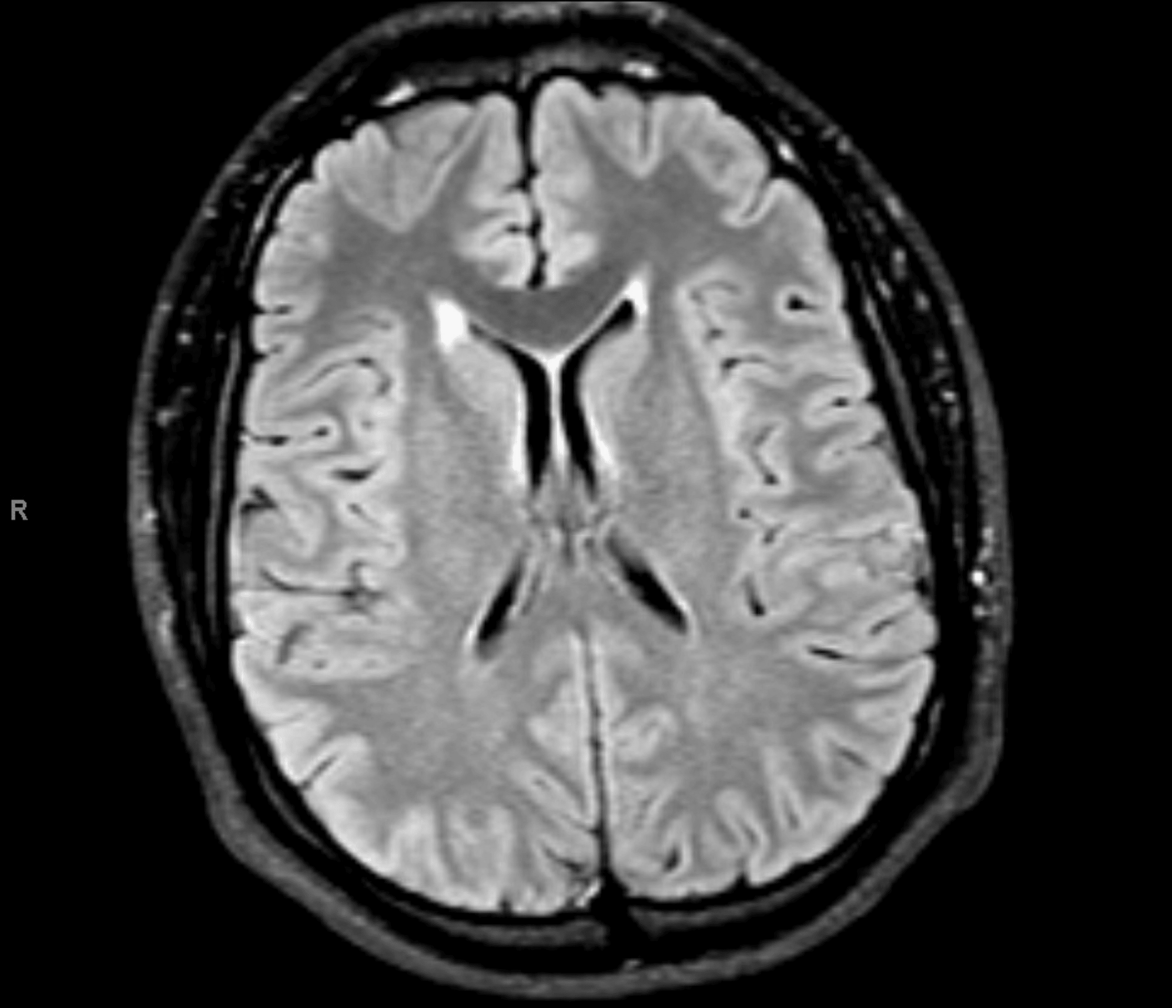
Brain MRI Imaging in a 22-year-old male with acute altered mental status and suspected NMS showed no evidence of acute ischemia, hemorrhage, mass lesions, or abnormal contrast enhancement. These findings reinforced the diagnosis of NMS by ruling out structural or vascular causes of his presentation and correlating with clinical signs of rhabdomyolysis and systemic inflammation. NMS: neuroleptic malignant syndrome
